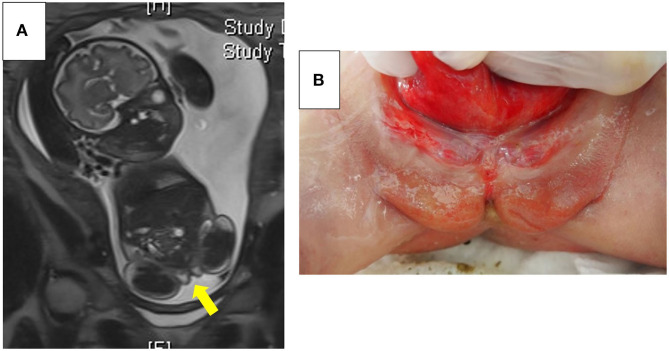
(A) Fetal magnetic resonance imaging in Case 2. A scrotum-like structure was noted (a yellow arrow). (B) Appearance of the external genitalia in Case 2. No apparent phallus or urethral meatus at the perineum was observed. Scrotum-like labioscrotal folds were noted. Permission to use the photo was granted by the parents.
